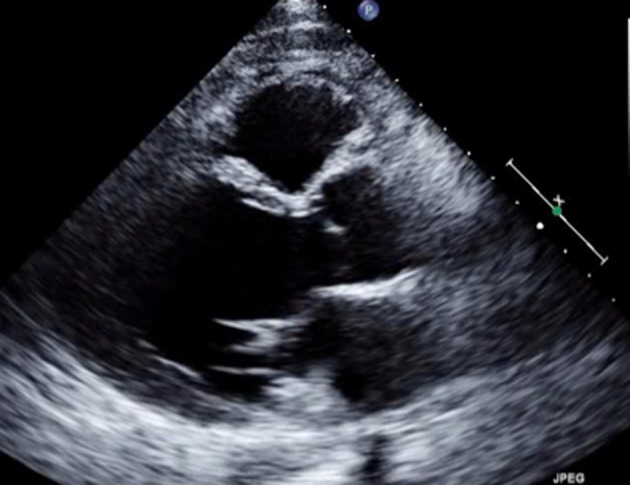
Echocardiogram with severe hypokinesis of anterior, anteroseptal walls, and apex.
